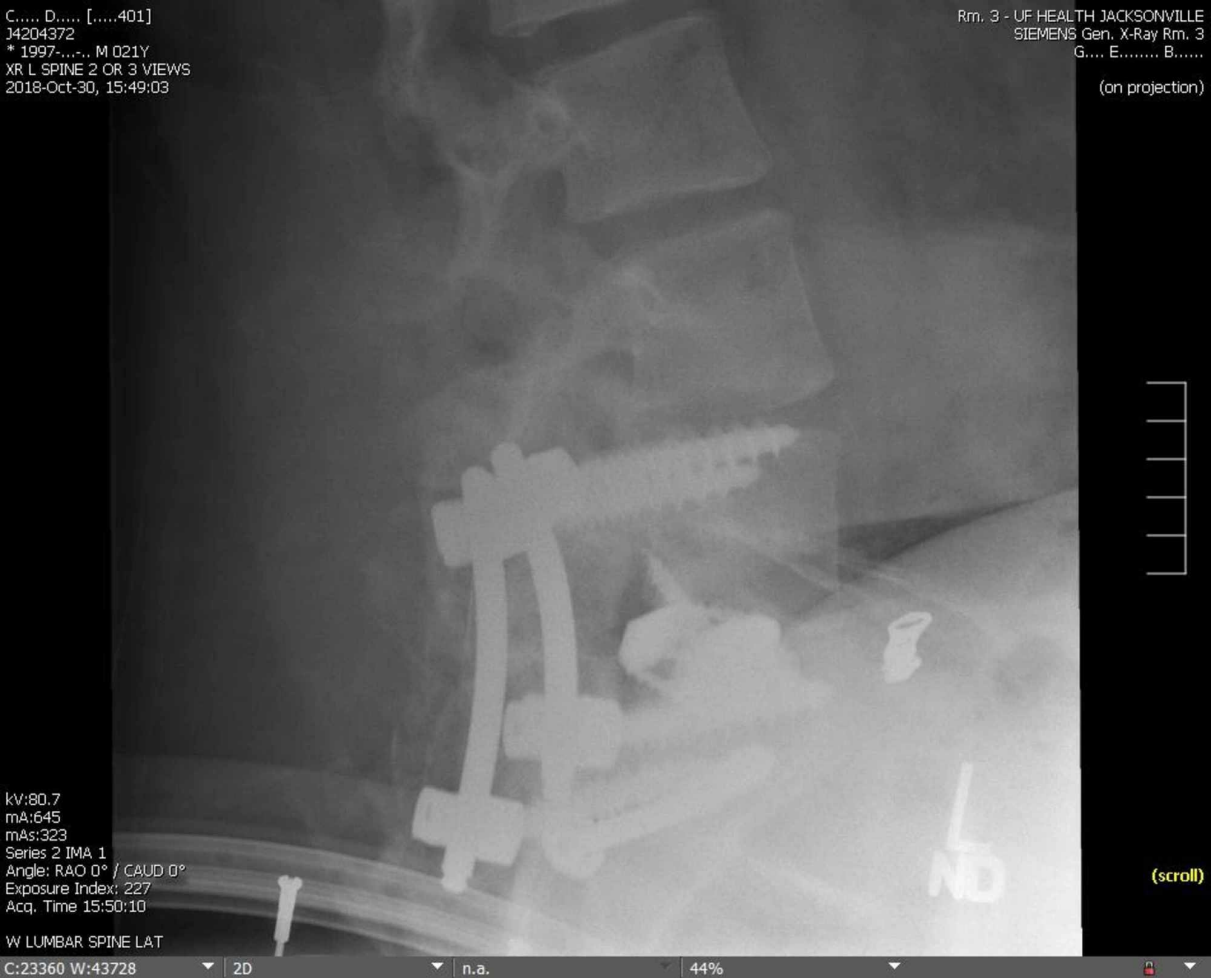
Lateral L-S X-ray at six months Postoperative X-ray revealing the anterior and posterior implant in a good position at six months
